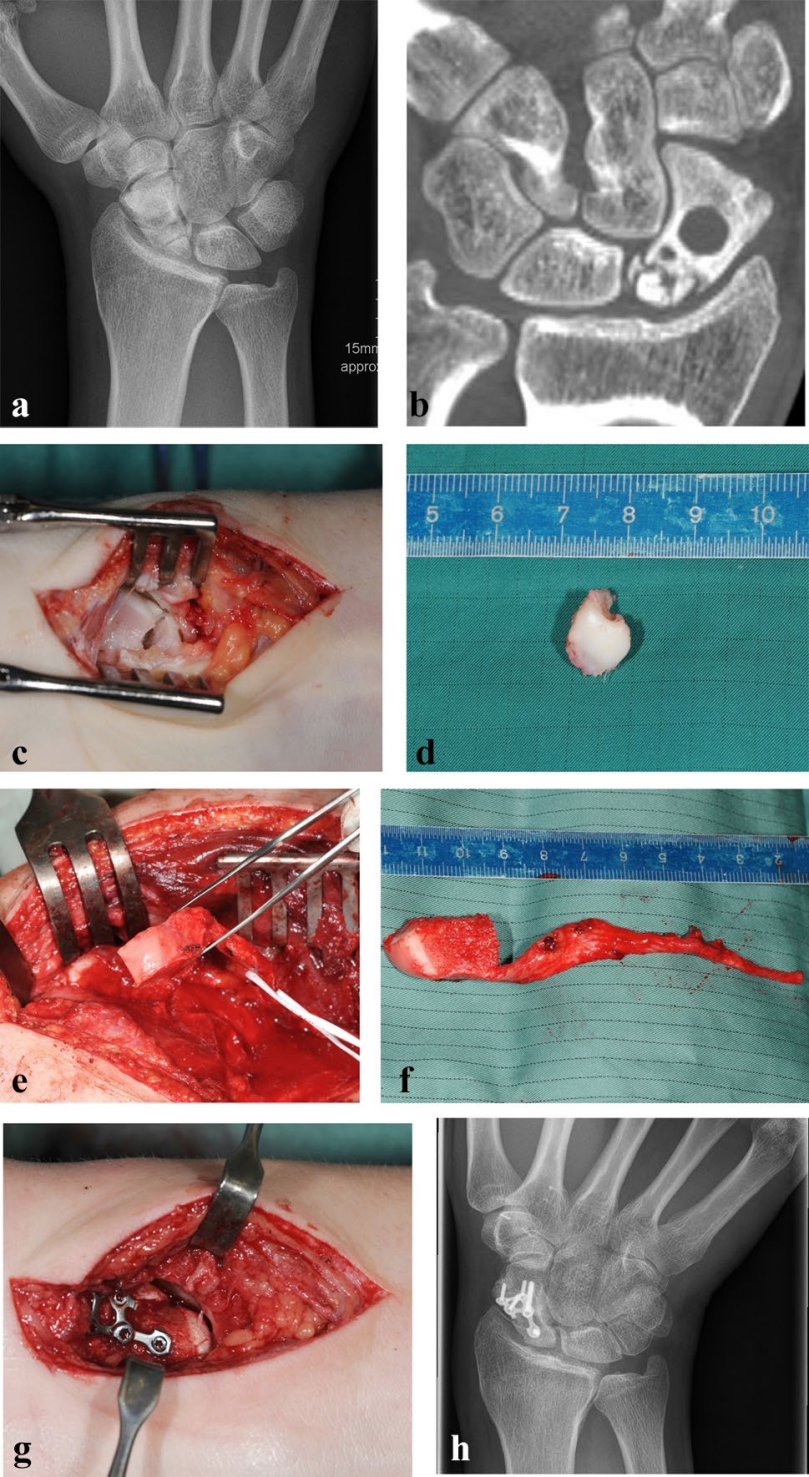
a Dorsopalmar radiograph of a 21-year-old man showing an established scaphoid non-union of the proximal pole. b Coronal CT scan showing the fragmented, necrotic proximal pole. c Intraoperative view showing the fragmented, necrotic proximal pole. d Intraoperative view showing the excised main proximal pole fragment. e Intraoperative view showing the harvesting of a osteochondral medial femoral condyle graft. f Intraoperative view showing the harvested osteochondral graft with a long pedicle. g Intraoperative view showing the replacement of the proximal pole with a osteochondral medial femoral condyle graft and plate fixation. h Dorsopalmar radiograph (3 years after surgery) showing the healed proximal pole without signs of carpal collapse
